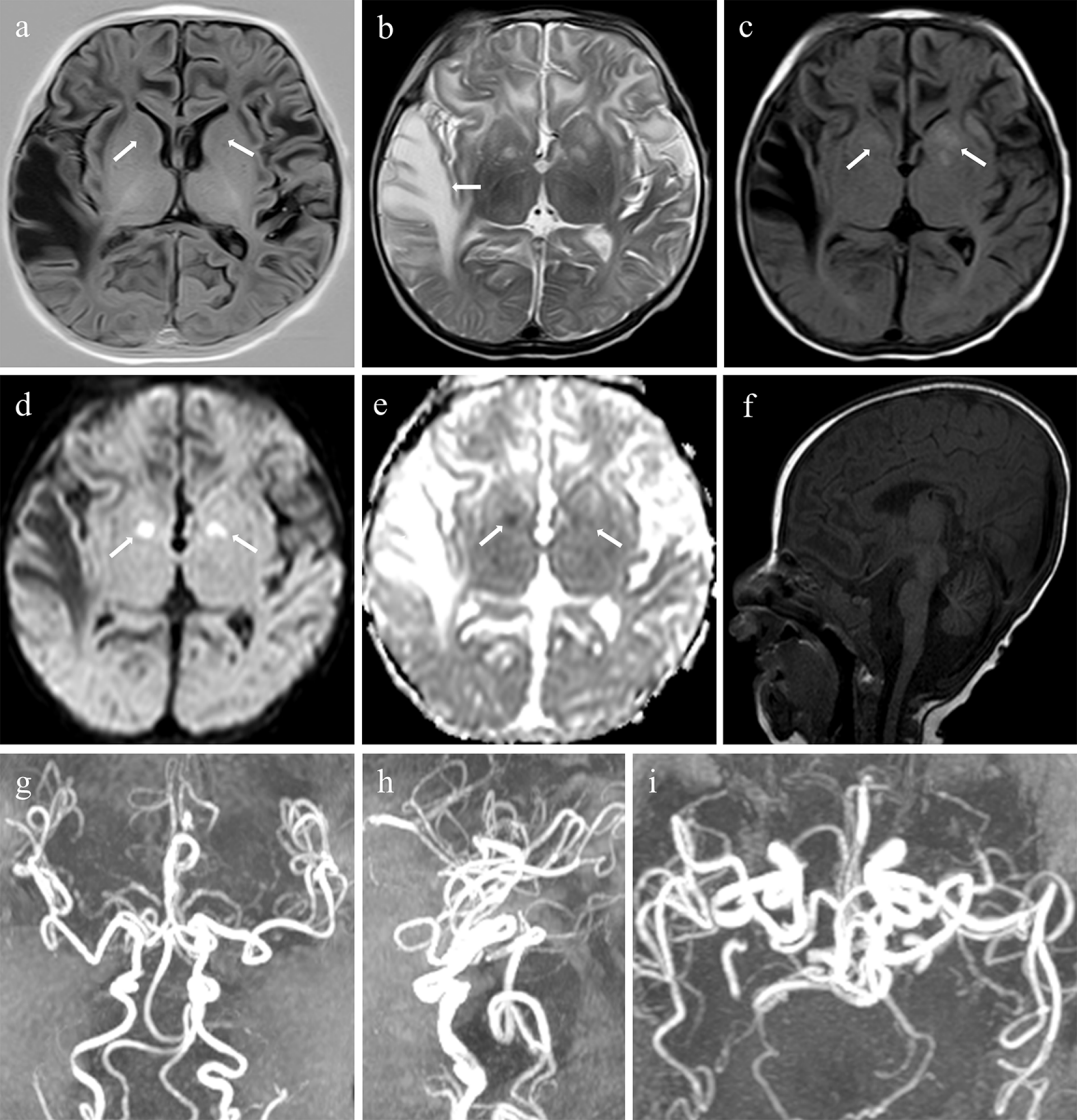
Cranial MRI images. (a-b) Axial T1-weighted image (T1WI) shows the delayed myelination (arrows). Axial T2-weighted image (T2WI) shows the distinctive tumefactive lesion in the right temporal lobe (arrow). (c-e) Axial T2-fluid-attenuated inversion recovery (T2-FLAIR) shows asymmetric hyperintensity in bilateral head of caudate nucleus and globus pallidus (arrows). Diffusion-weighted image (DWI) (b value = 800 s/mm2) and apparent diffusion coefficient (ADC) maps show asymmetric ovoid foci of restricted diffusion in bilateral globus pallidus (arrows). (f) Sagittal T1WI shows cerebellar atrophy. (g-i) Magnetic Resonance Angiography (MRA) shows increased tortuosity of the internal carotid, anterior cerebral, middle cerebral, posterior cerebral, vertebral and basilar arteries
